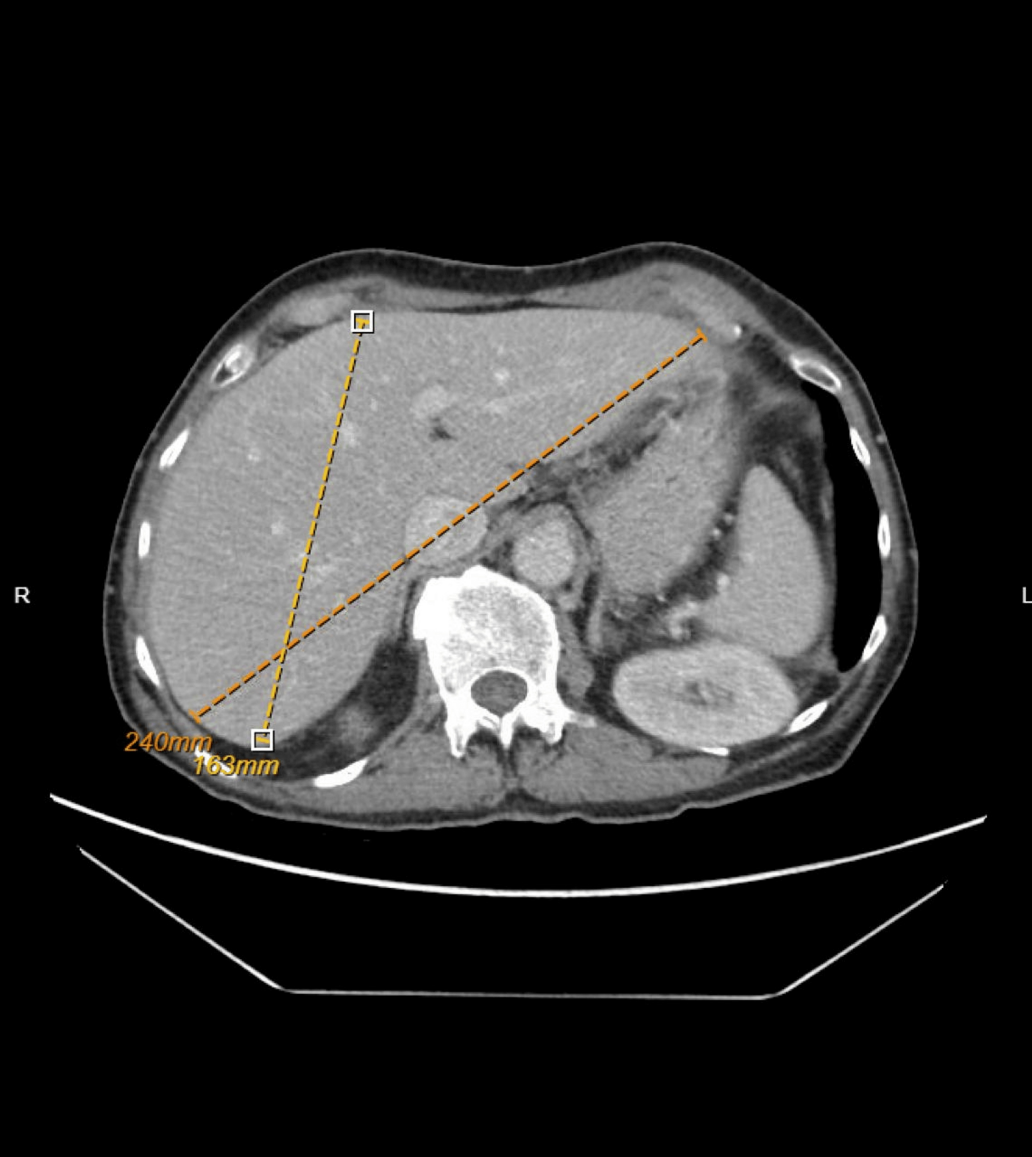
CTAP showing hepatic steatosis. CTAP, computed tomography arterial portography.
